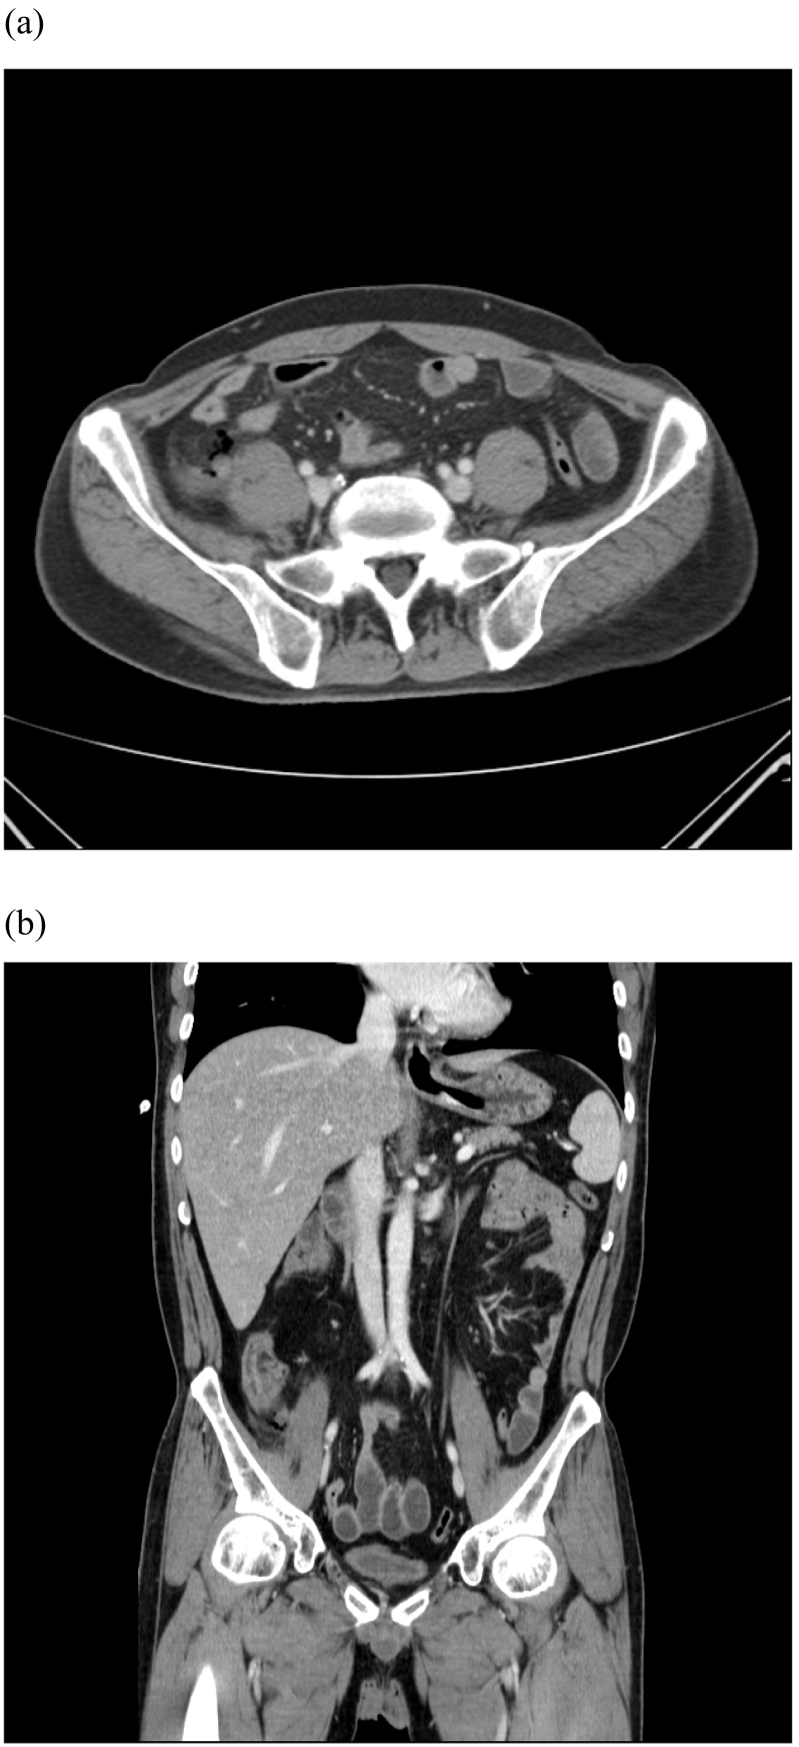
Contrast enhanced axial (a) and coronal (b) CT images at presentation shows perforated appendicitis with air bubble.
